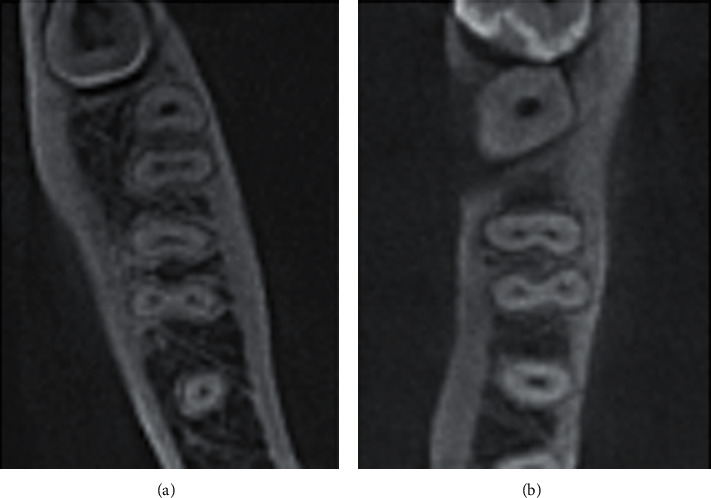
CBCT axial sections at midroots. (a) first and second mandibular molars with three canals and (b) first mandibular molar with four canals.
